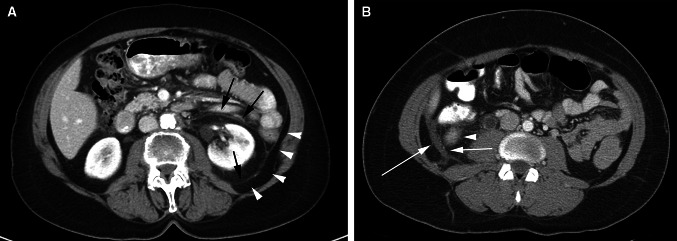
Renal fasciae. A Axial CT image shows the anterior and posterior renal fascia (*black arrows*). Arrowheads show the posterior pararenal space extending laterally as the properitoneal fat. B Axial CT image shows fluid (*arrows*) in between the 2 layers of the posterior renal fascia. Fluid from the anterior pararenal space can extend into this potential space between the 2 layers. *Arrowhead* points to the lower pole of the kidney.
